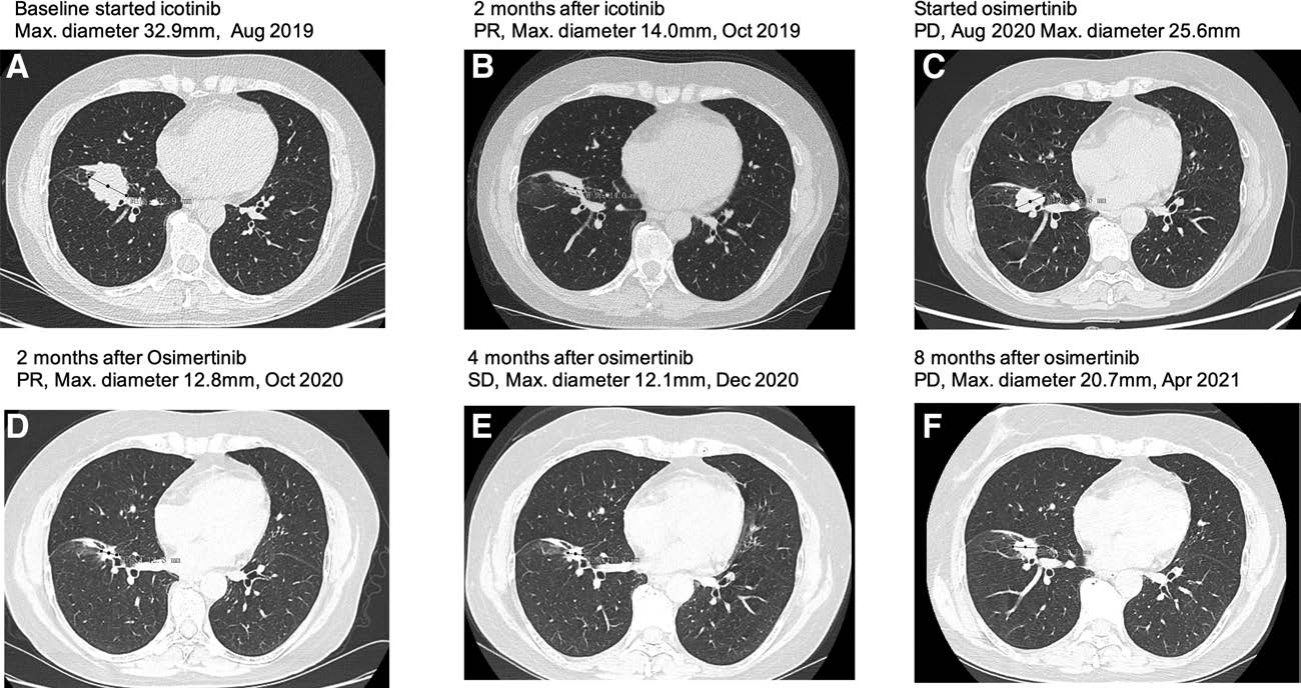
The CT images of the primary lung mass at baseline (A), at the evaluation of PR after 2 months of icotinib treatment (B), at the evaluation of PD after12 months of afatinib treatment (C), at the evaluation of PR after 2 months of osimertinib treatment (D), at the evaluation of SD after 4 months of osimertinib treatment (E), and at the evaluation of PD after 8 months of osimertinib treatment (F). CT = computed tomography, SD = stable disease, PR = partial response, PD = progressive disease.
